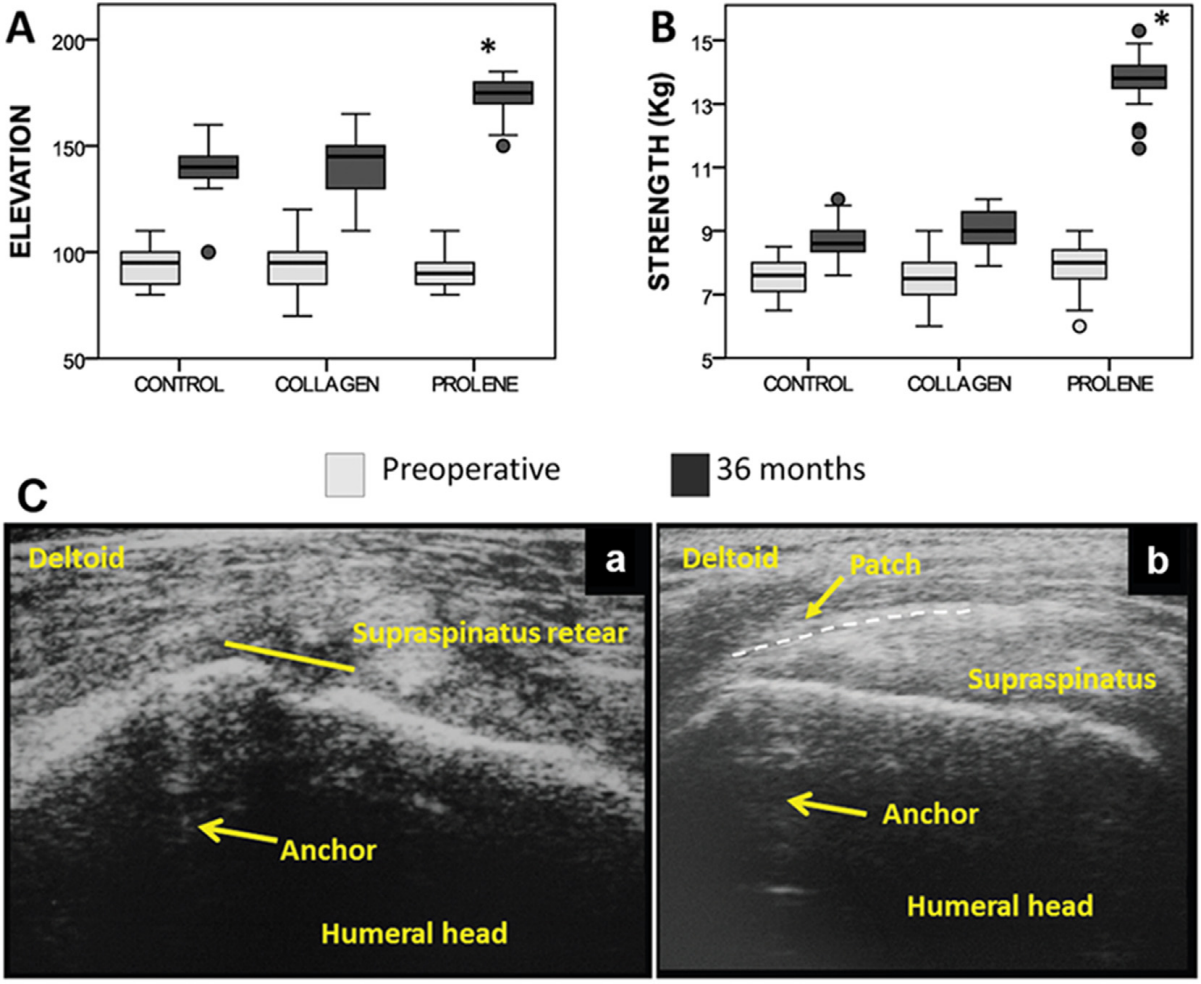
Shoulder function and tissue healing following open repair (control), collagen-mediated rotator cuff repair, and polypropylene (prolene) (Repol Angimesh®, Angiological BM Srl, Pavia, Italy) -mediated rotator cuff repair. (A) Boxplots summarizing elevation on the scapular plane; (B) abduction strength assessed pre- and 36 months post-operatively. ∗, Statistically significant difference at 36 months between the polypropylene group with other groups. Whiskers indicate minimum and maximum values. Round dots indicate outliers, defined as values higher than 1.5-fold the interquartile range. (C) Ultrasound images taken 12 months post-surgery showed (a) recurrent supraspinatus defect and collagen patch absorption, and (b) maintenance of rotator cuff integrity and the presence of a polypropylene patch. Copyright obtained from SAGE journals and adapted from Pietro Ciampi et al., 2014.
